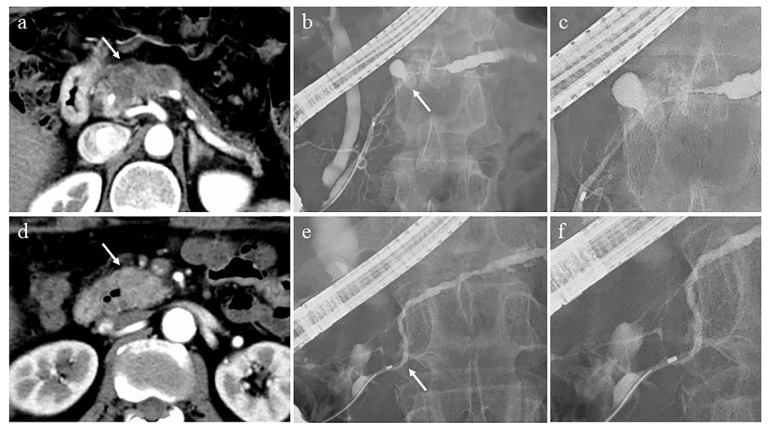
Cases of pancreatic cancer (a–c) and autoimmune pancreatitis (d–f). (a) Computed tomography (CT) shows an irregular and hypovascular 3 cm-seized mass in the pancreatic body (arrow) and dilatation of the upstream main pancreatic duct (MPD). (b) Endoscopic retrograde pancreatography (ERP) shows the MPD stricture in the pancreatic body (arrow) and upstream MPD dilatation. (c) Deviation of side branches from the stricture site is not observed. (d) CT shows a 2 cm-sized mass with hypovascularity in the arterial phase in the pancreatic head (arrow). (e) ERP shows mild strictures of the Wirsung and Santorini ducts (arrow), and slight upstream MPD dilatation. (f) Deviation of side branches from the stricture site is observed.
